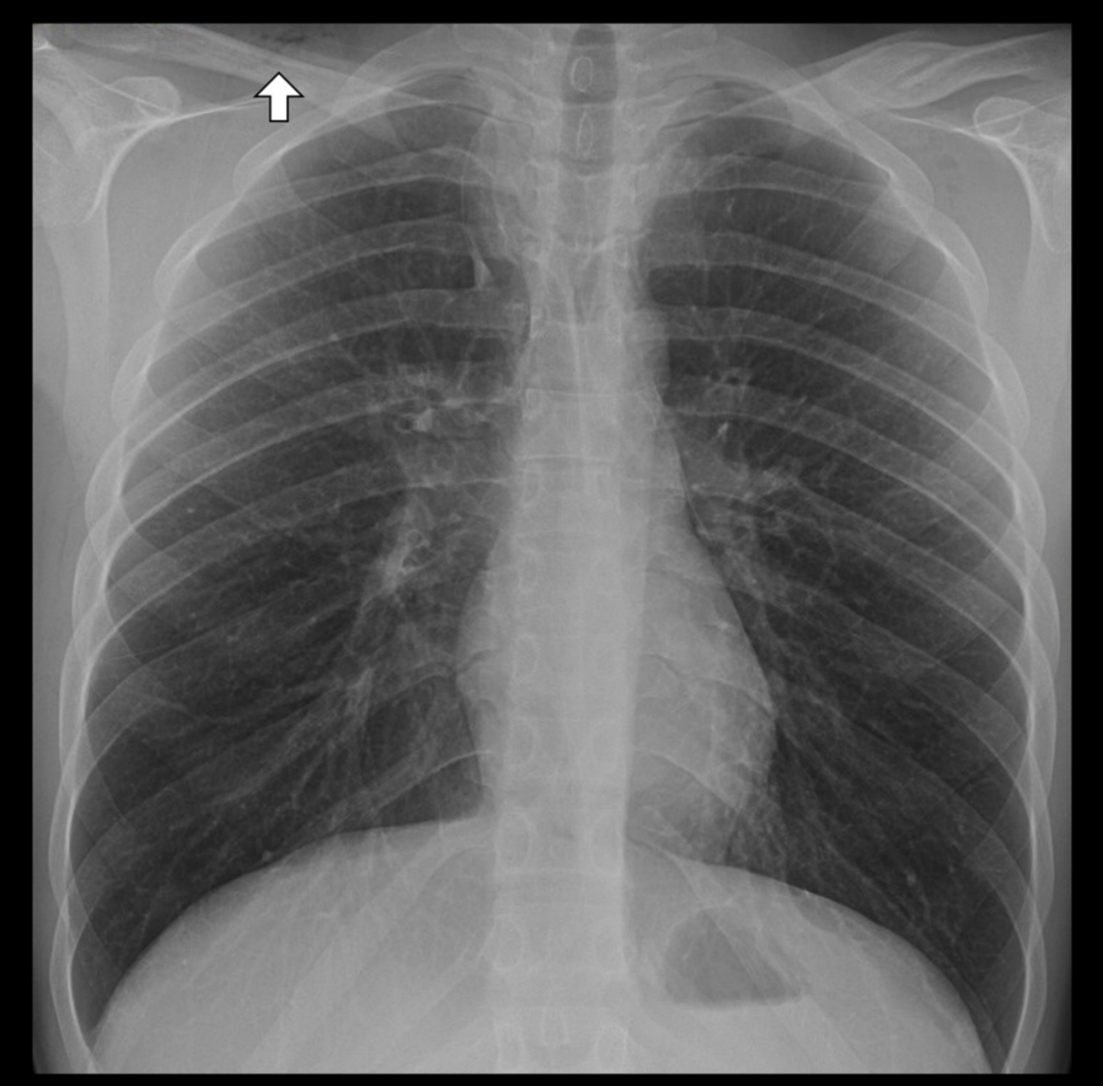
Plain film chest X-ray 12 hours after treatment with 12 liters per minute oxygen through a non-rebreather. Persistent pneumomediastinum with decreased soft tissue emphysema after treatment with high-flow oxygen.
